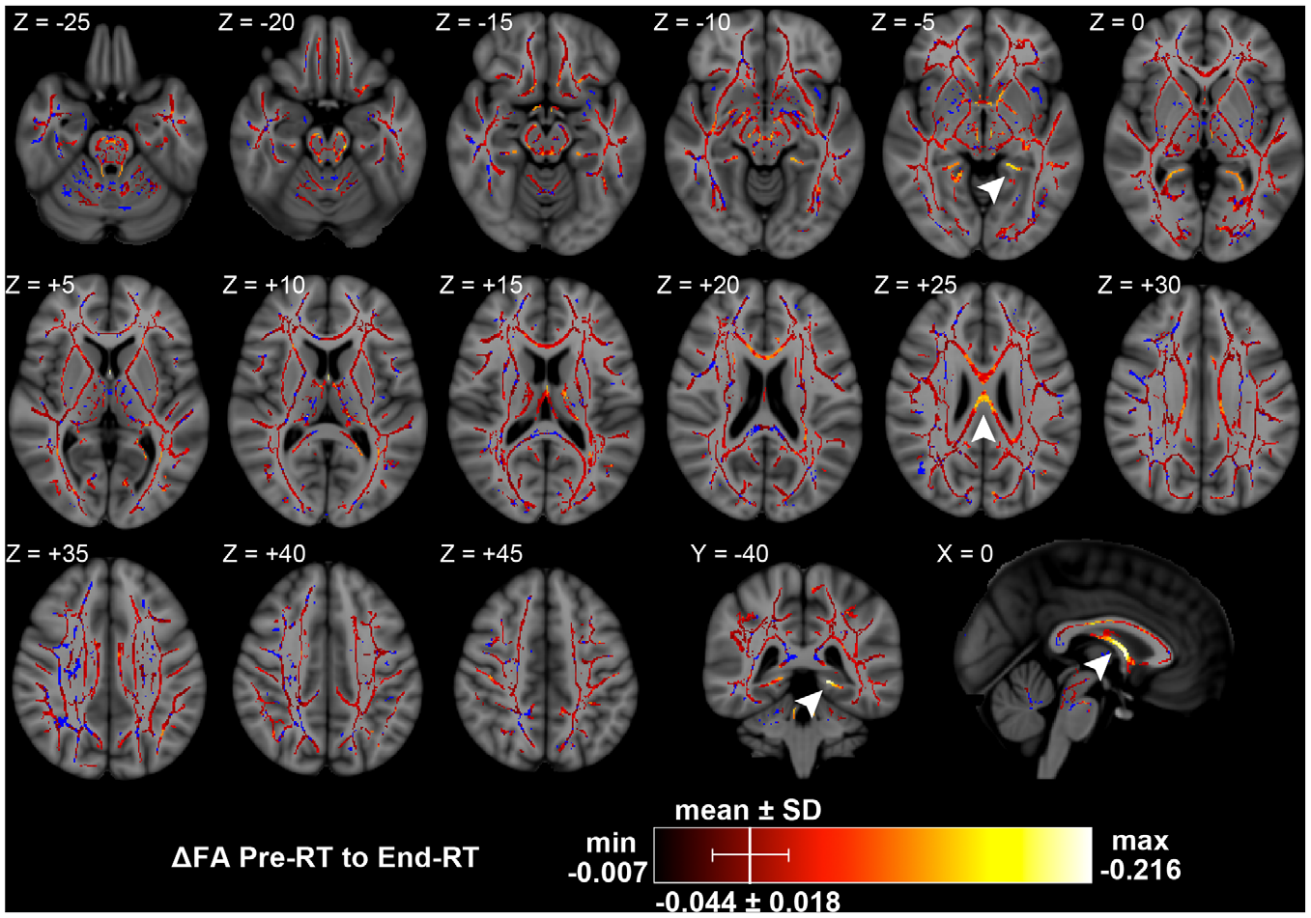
Significant changes in FA from pre-RT to end-RT. Arrowheads: Z = −5, left inferior cingulum; Z = +25, corpus callosum body; Y = −40, left inferior cingulum; X = 0, fornix columns. Significant results per color chart, blue is TBSS skeleton without significant results. Depicted on Montreal Neurological Institute (MNI) ICBM152 standard brain T1-weighted image [34].
